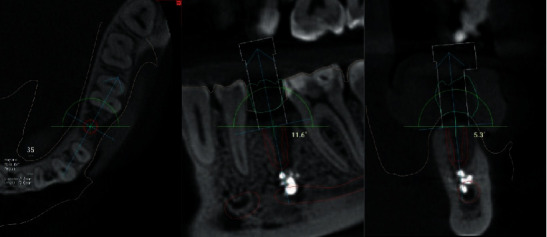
CBCT. The implant is installed virtually in the area of the missing 3.5 tooth. Fragments of filling material are visualized in the lumen of the mandibular canal.
